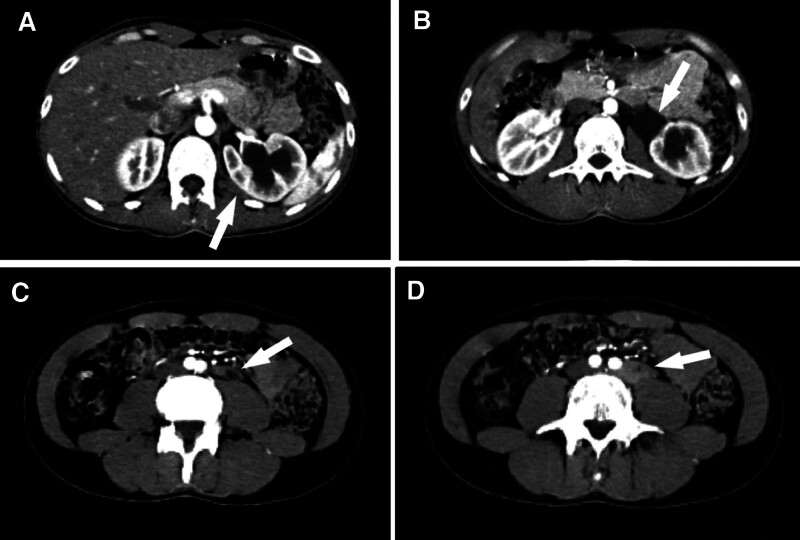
Axial contrast-enhanced computed tomography scans. (A) and (B) Hydroureteronephrosis of the left kidney with dilation of the pelvicalyceal system. (C) and (D) Homogenous lesion with uniform enhancement measuring 3.5 × 2 × 5.2 cm in the left retroperitoneum, adjacent to the left iliac artery, and encasing the ureter (indicated by arrows).
